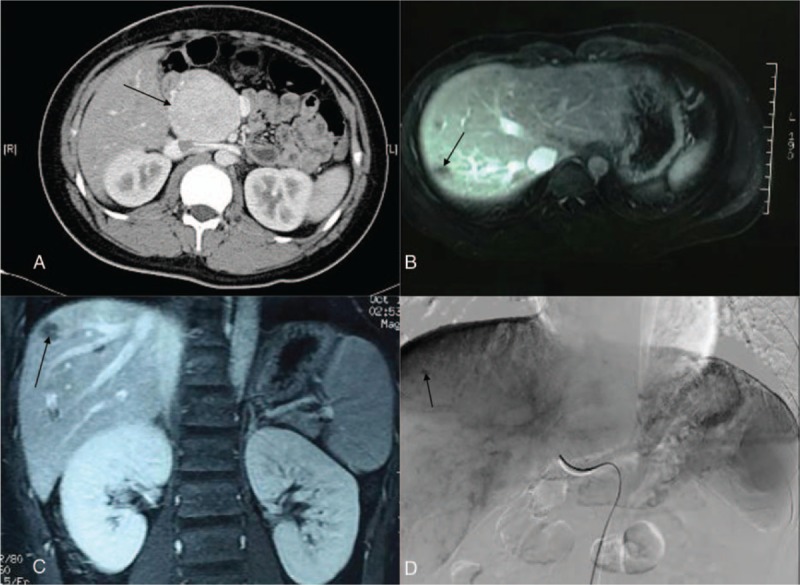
Preoperative images and liver metastasis images of patient 7. (A) Preoperative computed tomography scan showing a 7-cm lesion at the head of the pancreas. (B) Magnetic resonance imaging showing postoperative liver metastasis. (C) Magnetic resonance imaging of the coronary area showing postoperative liver metastasis. (D) Digital subtraction angiograph showing postoperative liver metastasis.
